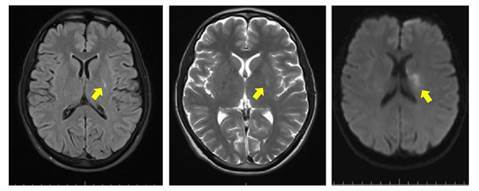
Brain magnetic resonance imaging: left: T1 sequence; middle: T2 sequence; right: diffusion sequence. White arrows show a small lesion (2.5 cm in size) with restriction to diffusion, compromising the left basal ganglia region, the caudate nucleus, and part of the corona radiata.
